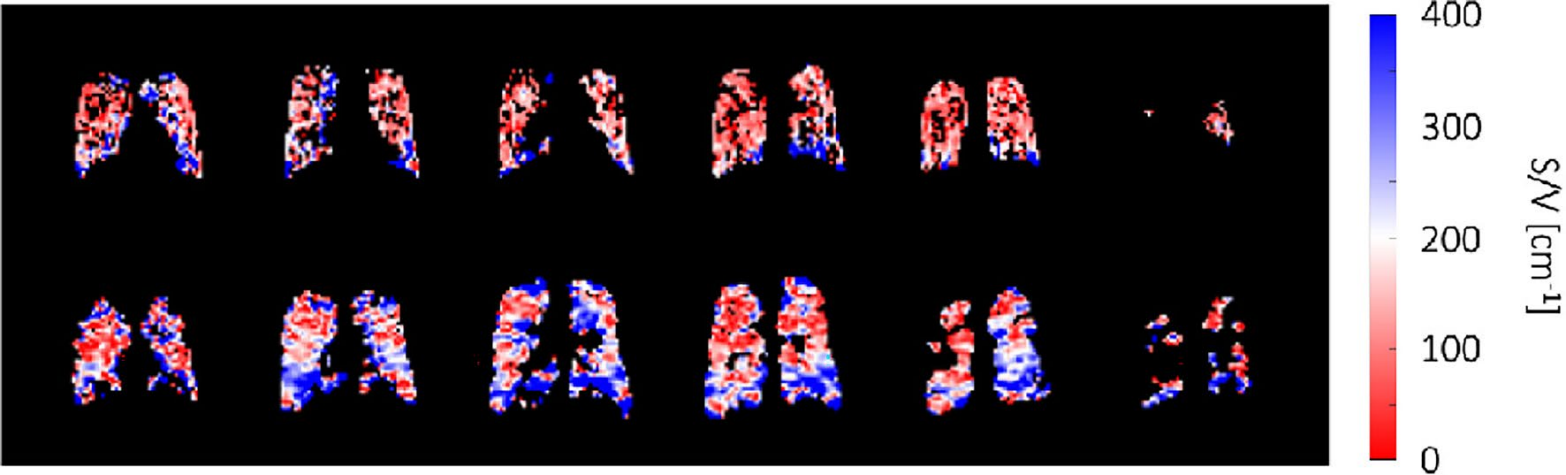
Measurement of the lung surface–volume (S/V) ratio using ^19^F (top row) and ^129^Xe (bottom row) diffusion‐weighted MR imaging at variable diffusion time in a patient with chronic obstructive pulmonary disease, forced expiratory volume in 1 s 63% of predicted value. Reproduced from [141], published under the terms of a Creative Commons Attribution License, https://creativecommons.org/licenses/by/4.0/.
